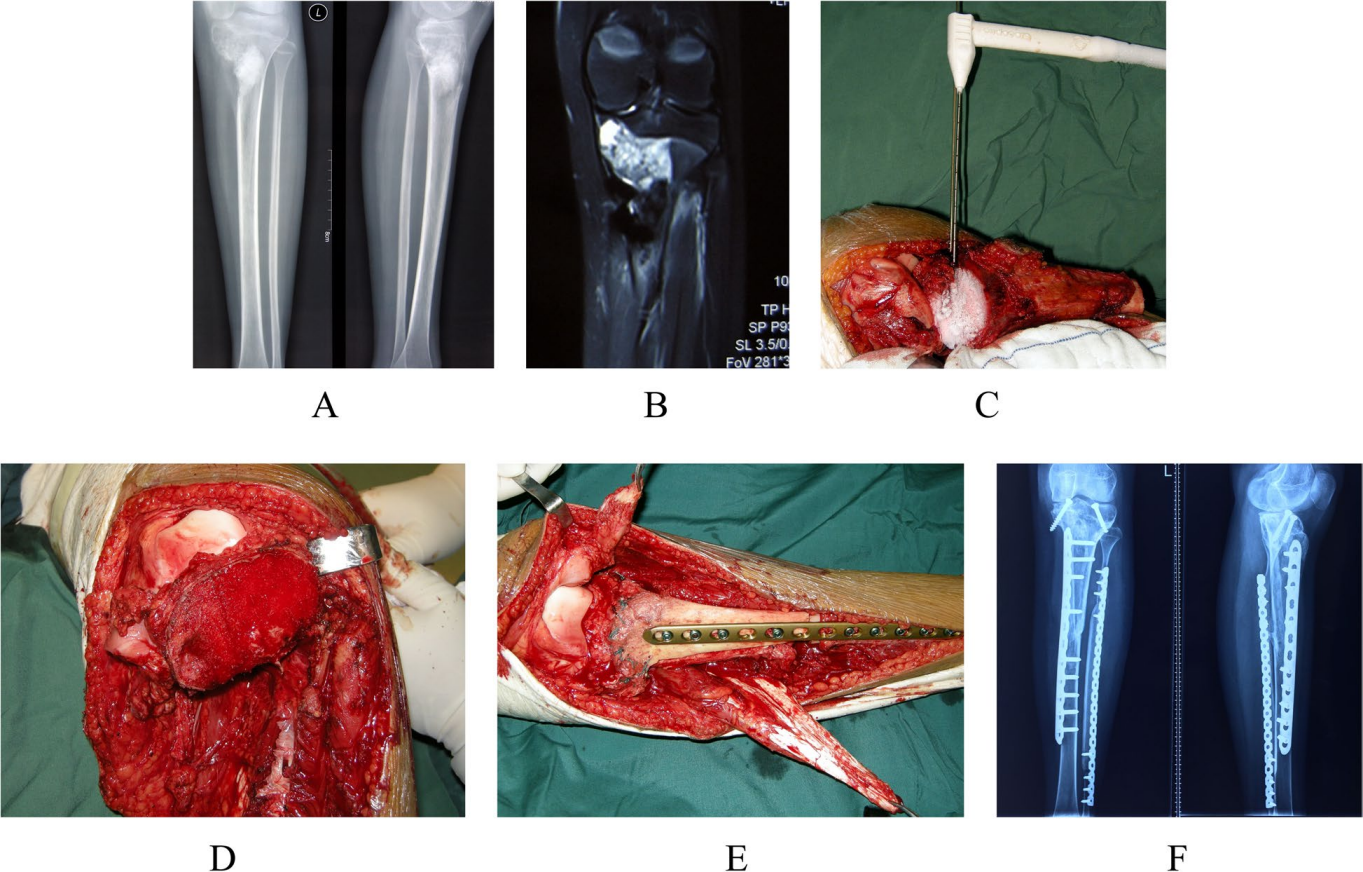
A–F A thirteen-year-old girl with an osteosarcoma in the proximal aspect of the tibia. A Anteroposterior radiograph showing tumor located at the proximal tibia. B Coronal magnetic resonance image showing the extent of tumor extension with epiphysis involvement. C An intraoperative view showing frosted medial plateau during in situ cryoablation. D An intraoperative view showing the preserved epiphysis after transepiphyseal osteotomy. The ablative bone margin was achieved in this case. E An intraoperative view showing intercalary reconstruction with recycled tumor-bearing bone and vascularized fibular graft. F Anteroposterior radiograph postoperative 54 months showing osteotomies healing with mature callus and mild degenerative change in the medial compartment of the knee. This patient had the MSTS score of 29
